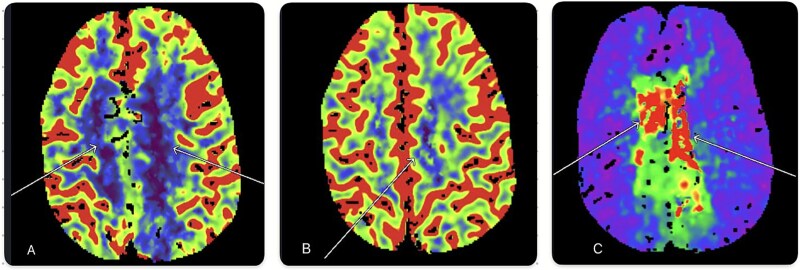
CT perfusion showing (A) reduced cerebral blood flow (CBF) in both anterior cerebral artery (ACA) distributions, (B) partial mismatch in cerebral blood volume (CBV), and (C) prolonged mean transit time (MTT). These findings suggest the presence of an ischemic penumbra.
